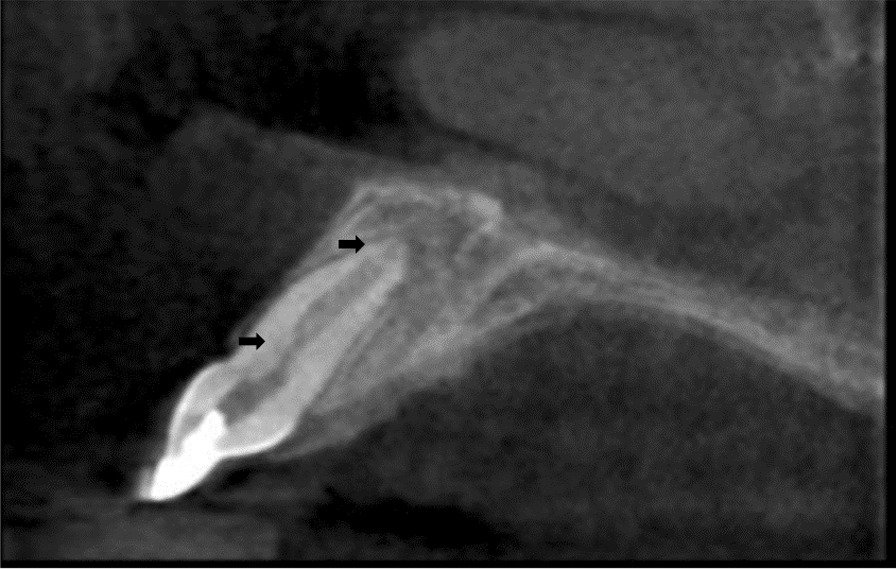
CBCT image at 12 months of 11 (three-dimensional apical closure)
